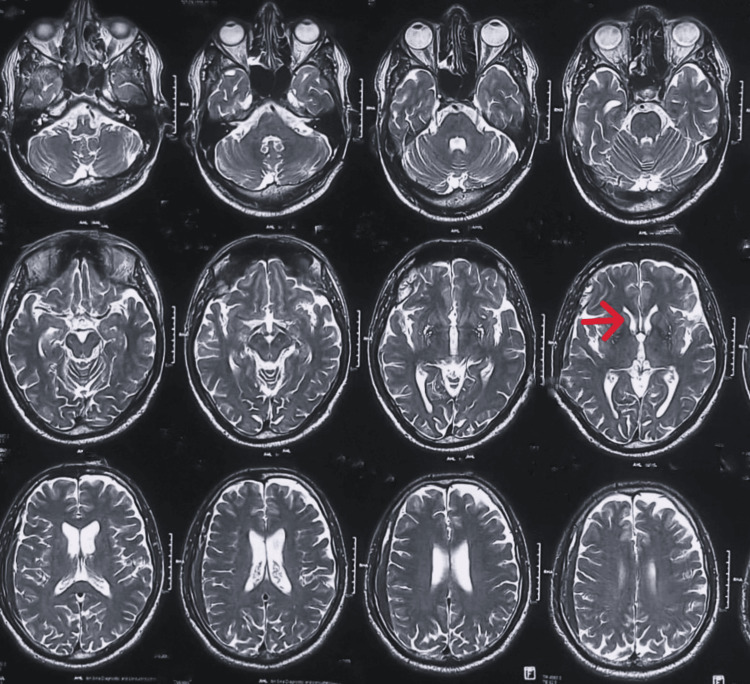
MRI of the brain (axial view) showing bilateral caudate nuclei atrophy.
